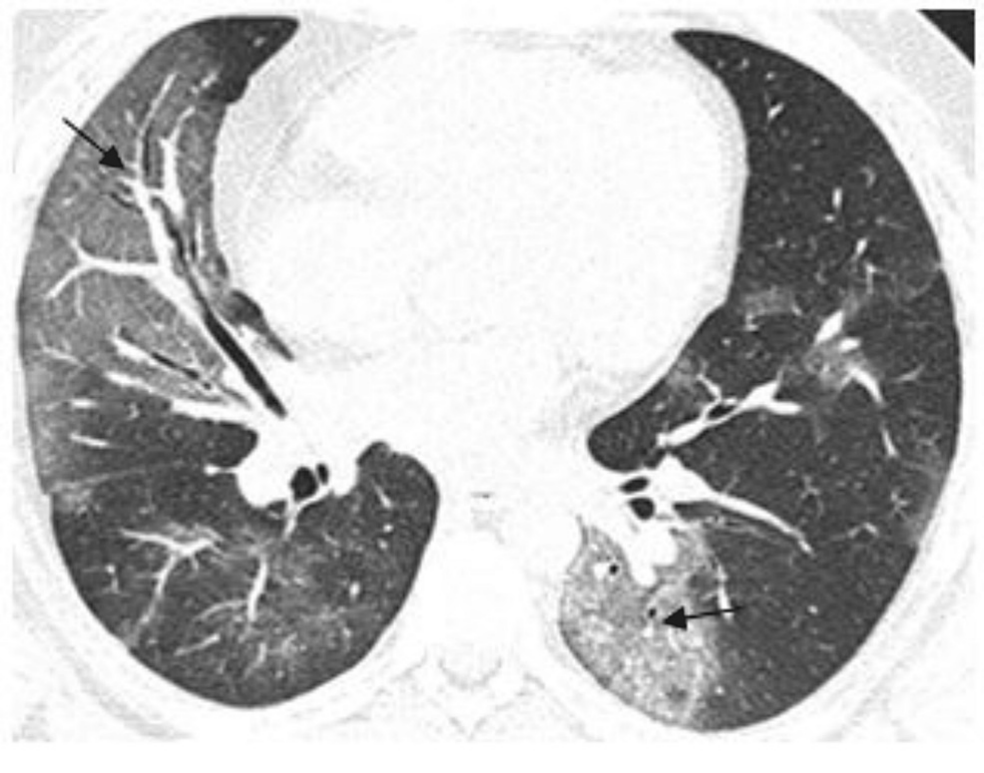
HRCT axial section of 38 Y/M COVID-19 positive patient showing ground-glass opacities with interlobular and intralobular septal thickening giving “crazy paving” pattern (arrows) in bilateral lung parenchyma.
